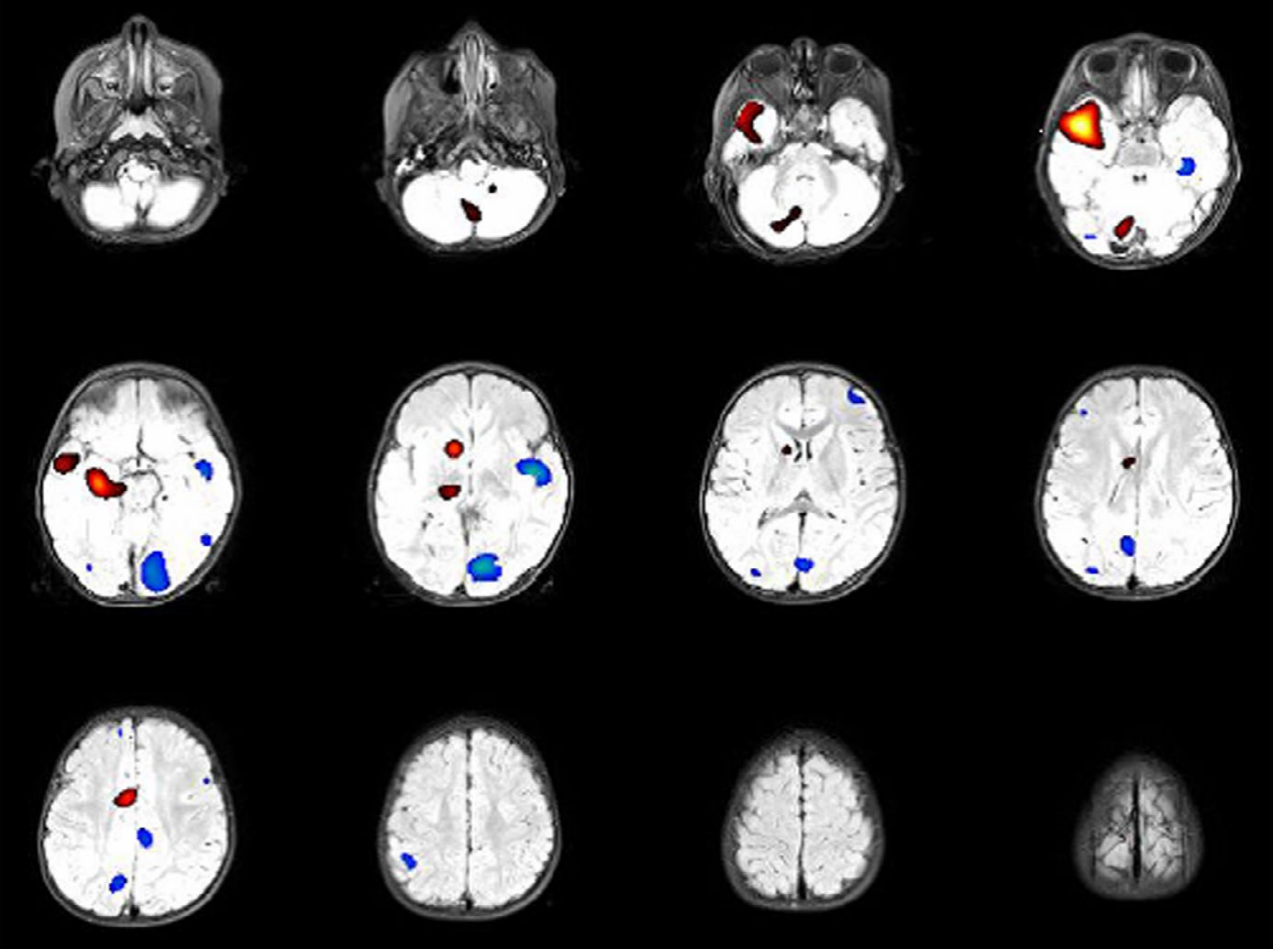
Subtraction ictal SPECT coregistered to MRI (SISCOM) imaging. Relative ictal hyperperfusion (+2 to +4 SD) is identified in red‐yellow, while the relative ictal hypoperfusion zones are colored in blue (−2 to −4 SD). Notice the localized zone of relative hyperperfusion in the right anteromesial temporal lobe (yellow), with propagation to the superior lateral temporal neocortex (red), while the contralateral anteromesial temporal lobe shows a light hypoperfusion (light‐blue). The patient reached complete seizure freedom after resective surgery, which confirmed the presence of a focal cortical dysplasia type IIA in the right anterior temporal lobe (patient #39)
